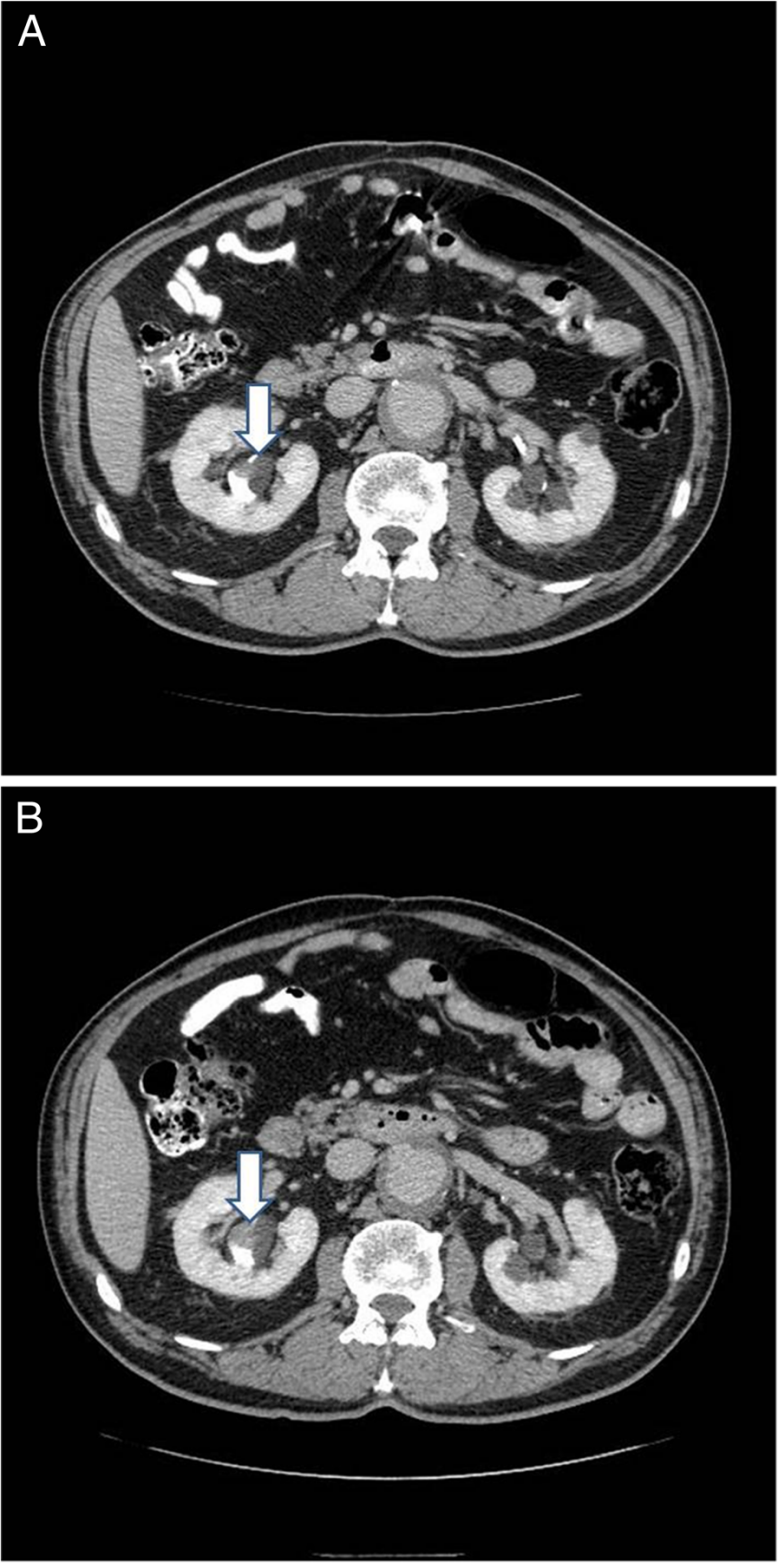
CT scans indicating the presence of the tumor (arrow) from (A) September 2010 and (B) June 2011.
